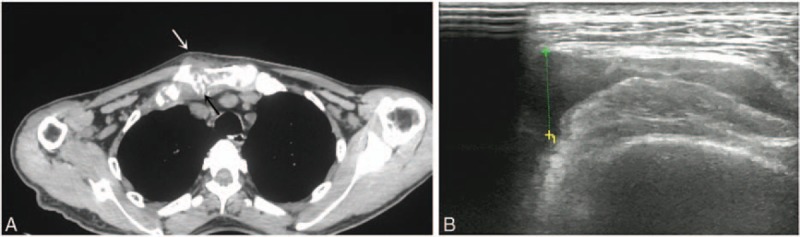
(A) Computed tomography (CT) radiograph showing bone destruction (black arrow) and soft tissue swelling (white arrow) in the right sternoclavicular joint. (B) Ultrasonogram showing effusion in the knee joints.
